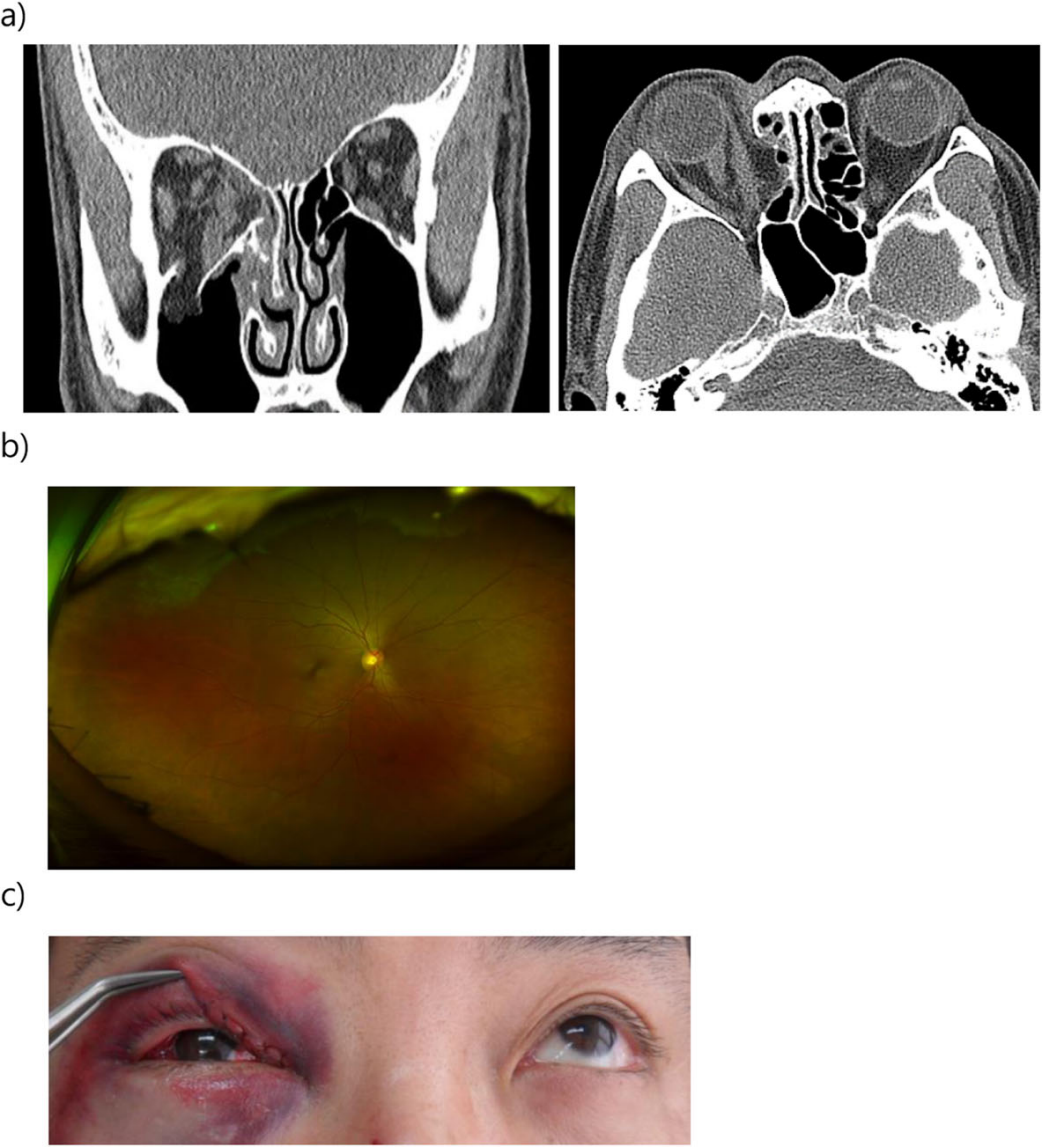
Preoperative CT images, fundus photograph, and eyelid photo. a Coronal and axial section showing fracture of the right superior/inferior/medial orbital wall and entrapment of the right inferior rectus muscle with orbital fat herniation to the right maxillary antrum. b Fundus photograph showing non-specific sign. c The limitation of the upward gaze is demonstrated
